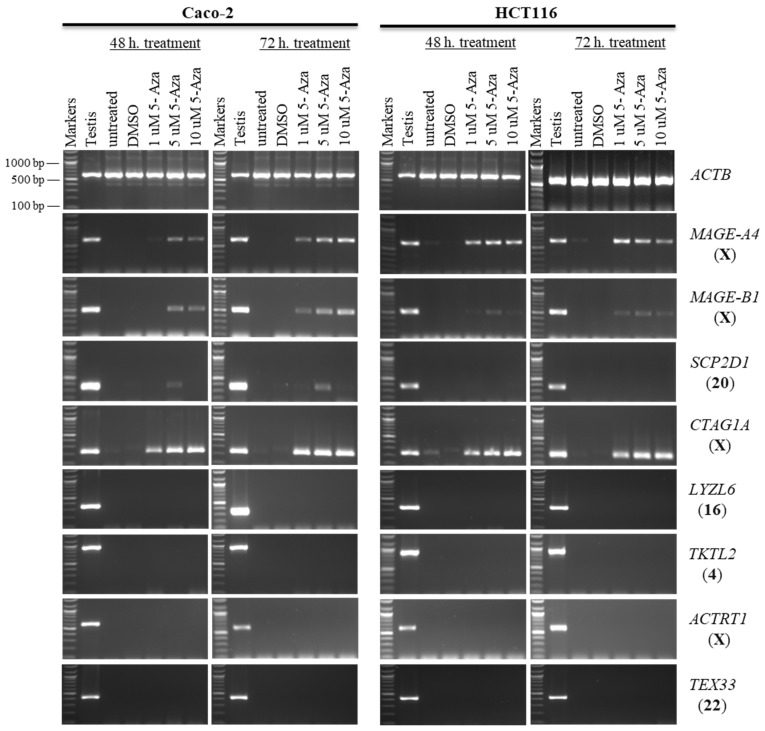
The effects of 5-aza-CdR treatments on CT gene expression profiles in the Caco-2 and HCT116 cancer cell lines. The expression of *SCP2D1*, *CTAG1A*, *LYZL6*, *TKTL2*, *ACTRT1*, and *TEX33* genes is shown on agarose gels following treatment with various doses of 5-aza-CdR for 48 h (left column of each cell) or 72 h (right column of each cell). Untreated Caco-2 and HCT116 cells were utilized as controls to compare the expression of CT genes with treated cells, and a testis sample served as a positive control for primer efficiency. The control Caco-2 and HCT116 cells were treated with DMSO, as DMSO was the solvent used in the 5-aza-CdR solution. The positive control for the cDNA samples is the expression of the *ACTB* gene. The official names of the genes are written to the right of the agarose gel images, and the location of each gene on the chromosomal is written in parentheses on the right. Above each lane, the particular concentration of 5-aza-CdR (1, 5, and 10 µM) is written.
